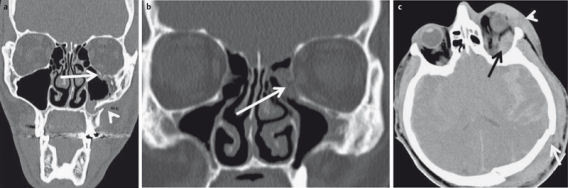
CT of the midface for detection of orbital fractures. a) 23-year-old football player with direct trauma to the left face and new onset of diplopia. Fracture of the floor of the orbita (arrow) with herniation of extraconal fat. b) 41-year-old patient with direct trauma to the globe. Fracture of the medial wall of the orbita and herniation of the medial rectus muscle (arrow). c) 86-year-old patient with severe head injury and rapid onset of exophthalmos. Intraorbital extraconal hematoma (black arrow) and periorbital hematoma (arrowhead). Impression fracture of the skull (white arrow).
